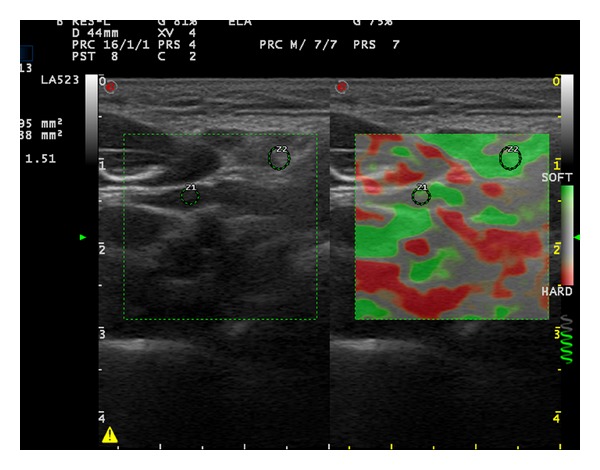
Elastosonography of the cervical lymph node on the right side of a 36-year-old male patient (long axis: 16.1 mm, short axis: 4.4 mm, cortical thickness: 1.3 mm, hilar thickness: 1.9 mm, strain ratio: 1.51, and elasticity score: 1).
